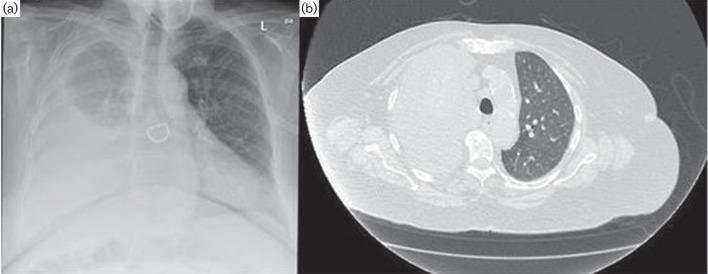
Patient’s chest X-ray (a) and CT scan (b) showing a large right-sided pleural effusion with lung atelectasis.
